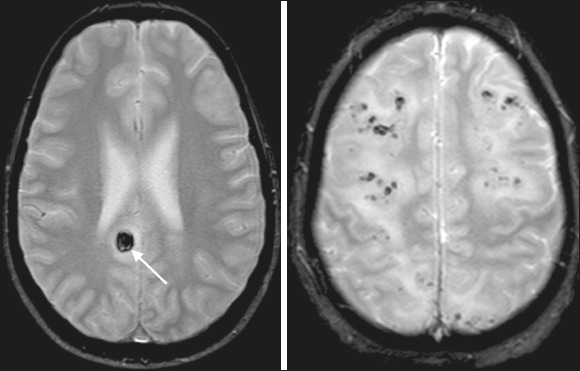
Brain MRI. Axial T2*-weighted sequence showing lesions containing hemorrhagic
